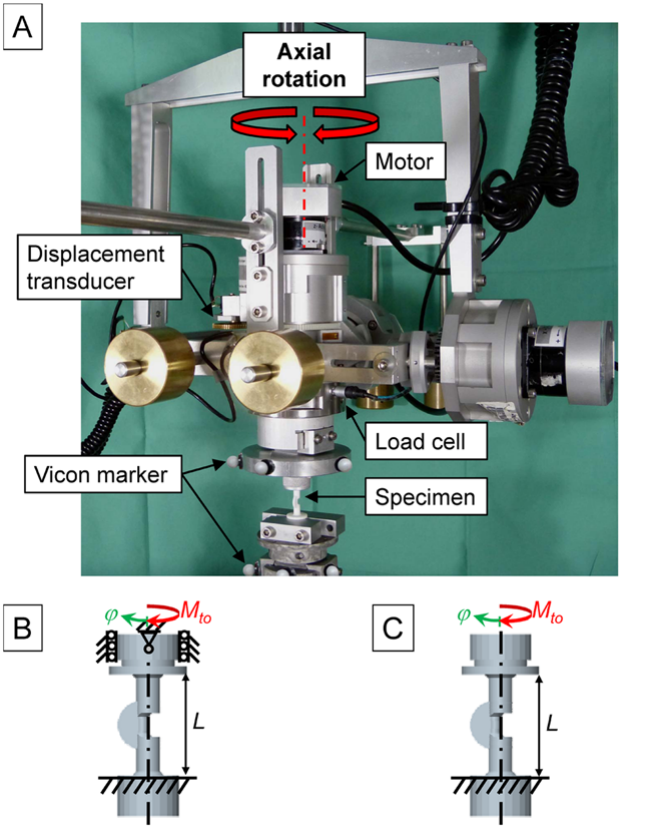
Torsional testing. A) Test set-up for torsional testing in the multi-axial flexibility testing machine. B) boundary conditions for constrained torsional testing (CT) and C) boundary conditions for the unconstrained torsional testing (UT).
